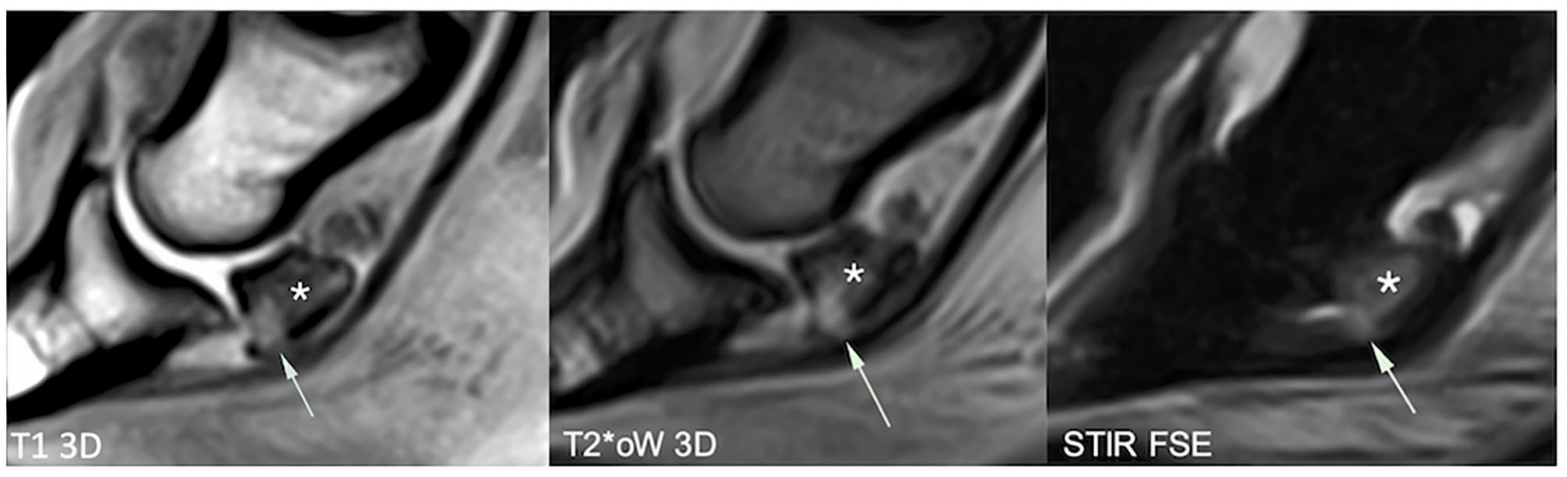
Sagittal magnetic resonance (MR) images focused on the navicular bone. There is a distal palmar compact bone erosion, characterized by disruption of the palmar compact and hyperintense signal intensity in all MR sequences, penetrating the adjacent palmar spongiosa (arrows). The navicular bone also shows extensive T1 hypointensity, STIR hyperintensity, and mixed T2*oW signal in the distal two-thirds of the palmar spongiosa, consistent with bone edema-like signal (asterisk). T1 3D, tridimensional gradient echo T1 weighted sequence; T2*oW 3D, tridimensional out of phase gradient echo T2* weighted sequence; STIR FSE, fast spin echo short tau inversion recovery weighted sequence; US, Ultrasonography.
